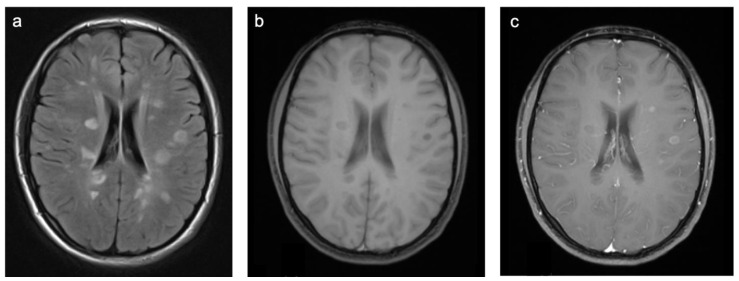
Representative case “Autoimmune disorders”: MS. FLAIR (a), T1W pre- and post-contrast (b,c) sequences. Fifteen-year-old girl diagnosed with MS. MRI shows characteristic ovoid/round lesions with asymmetric distribution abutting the lateral ventricles (periventricular) and touching the cortex (juxtacortical) [19] (a). Contrast-enhancing lesions in T1W post-contrast sequences are considered markers of blood–brain barrier breakdown (c).
